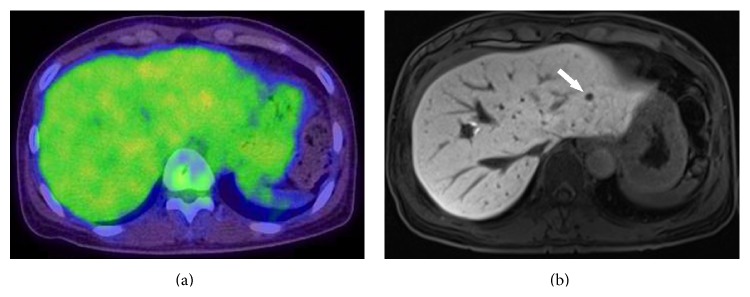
A 51-year-old woman with sigmoid colon cancer, who showed liver metastasis only on Gadoxetic acid-enhanced liver MRI. (a) Axial ^18^F-FDG-PET/CT image shows inhomogeneous physiologic activity of liver but no definite metastatic nodule. (b) Axial image of hepatocyte-phase on liver MRI shows a focal signal defect in segment 2 suggesting metastatic nodule (arrow).
